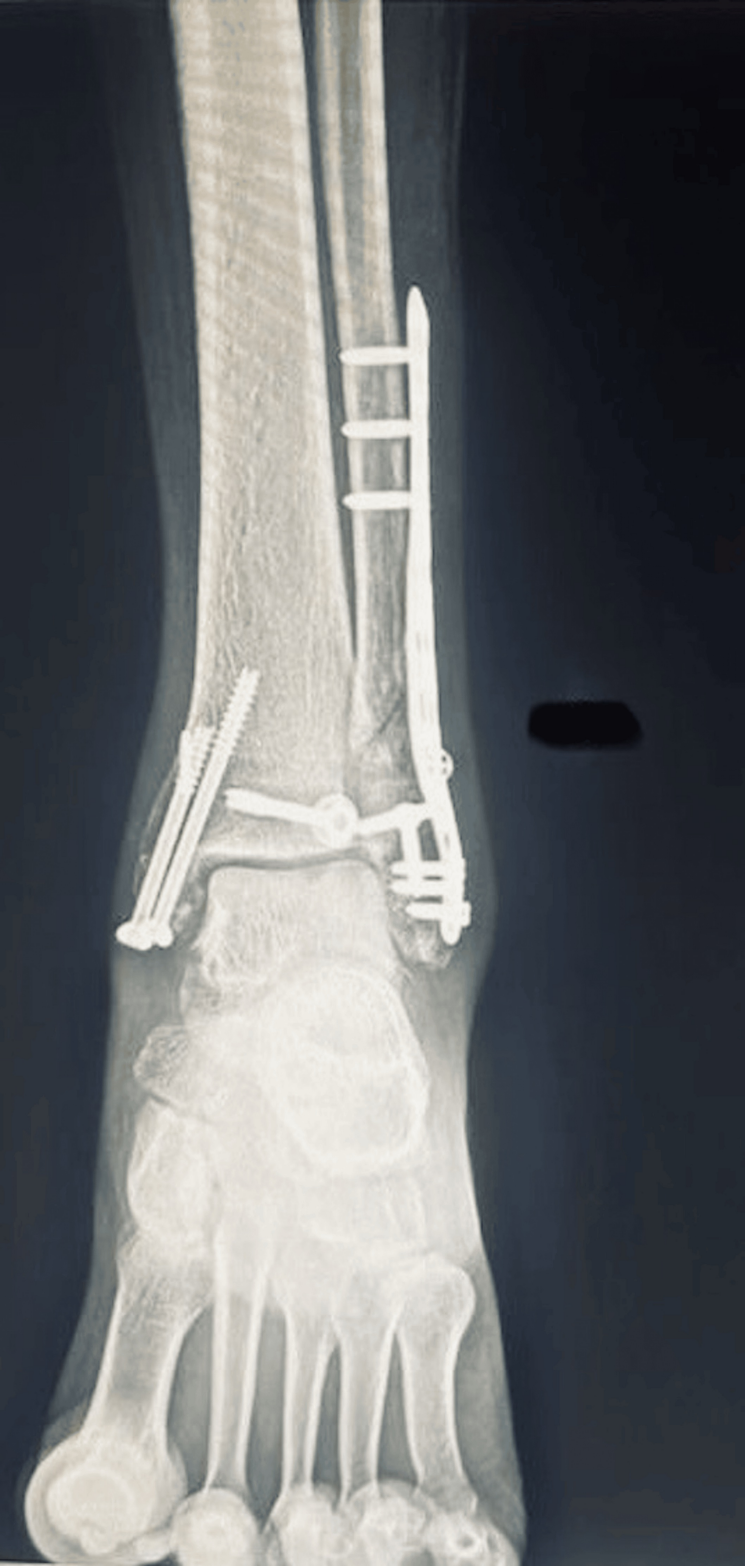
Post-operative X-ray (anteroposterior (AP) view) of the left ankle
